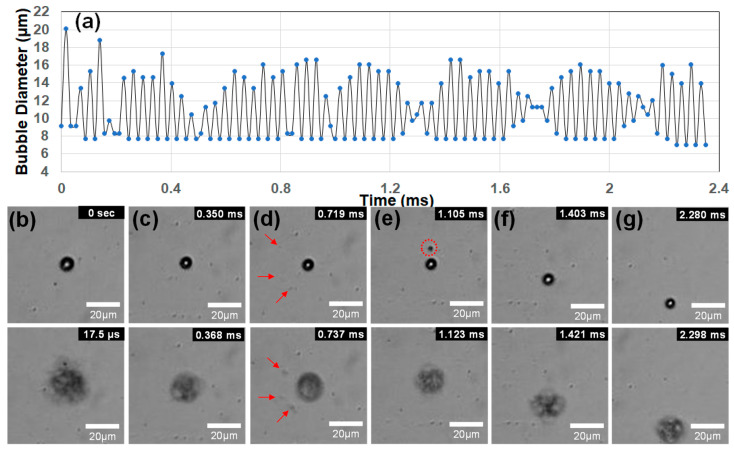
(a) A single lipid-DDFP droplet with an initial diameter of 9.17 µm exhibited 62 consecutive vaporization–condensation cycles using 1.7 MHz ultrasound and a sampling time of 17.54 µs at 24 °C. (b–g) Six vaporization–condensation oscillation images of the droplet sample. After the first vaporization, the bubble size decreased slightly with increasing cycles. Bubble fragmentation was observed to occur due to the expanding and shrinking oscillations in (d,e). The three droplet fragments in (d), indicated by the red arrows, expand individually without merging back into the main droplet. However, a single fragment in (e) does merge during the subsequent vaporization.
